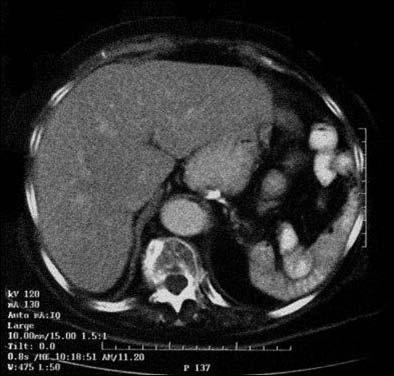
A CT scan of the patient one year after surgery.
